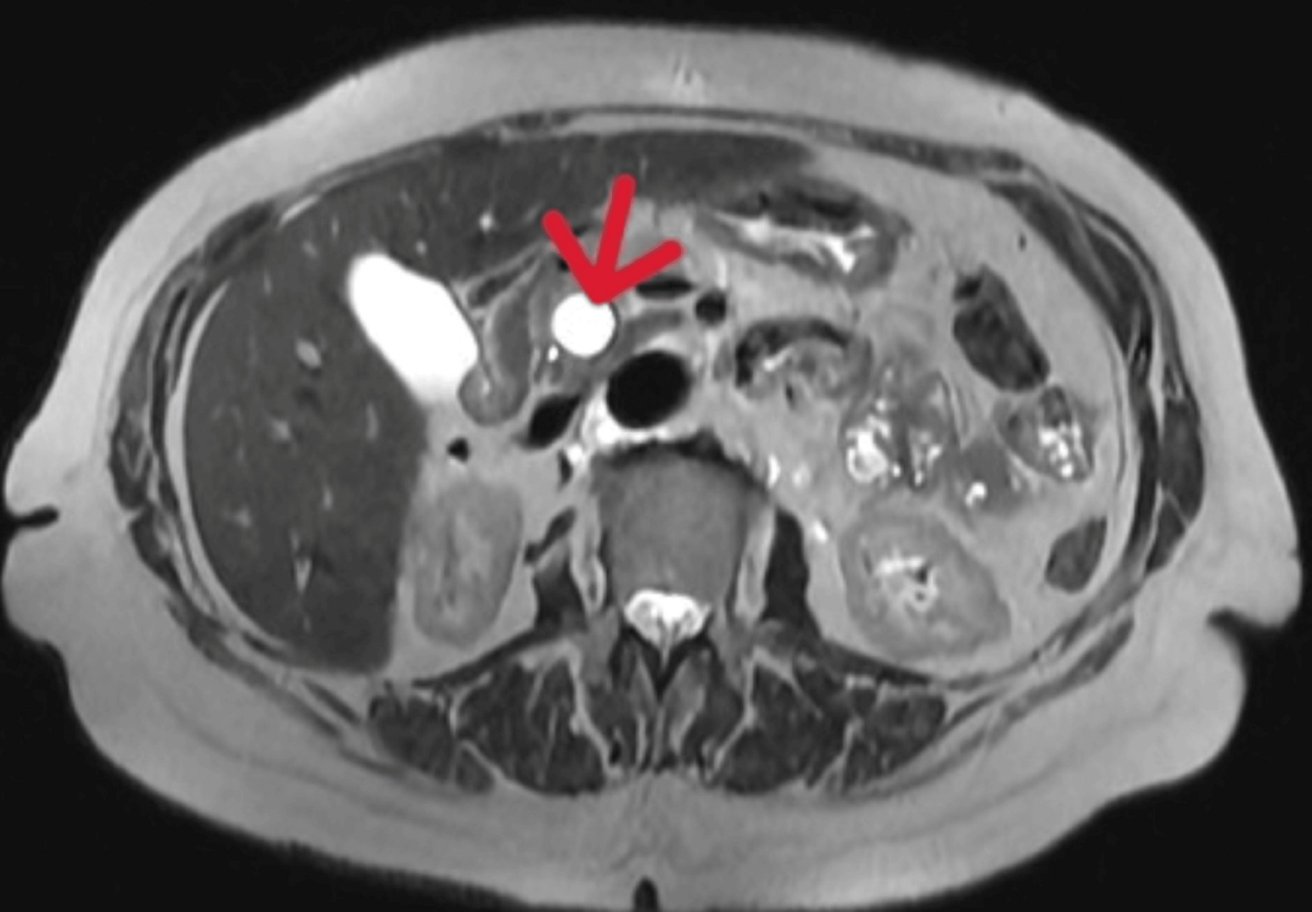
Axial MRI T2-weighted SSFSE sequence. The arrow indicates a well-defined cystic lesion in the pancreatic head. SSFSE: single-shot fast spin-echo
